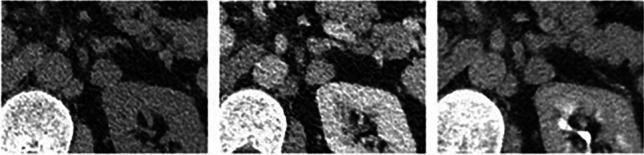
Axial CT images of a left adrenal nodule with benign washout characteristics, later proven to be malignant phaeochromocytoma following resection; left—non-contrast phase, middle—portal venous phase and right—delayed
